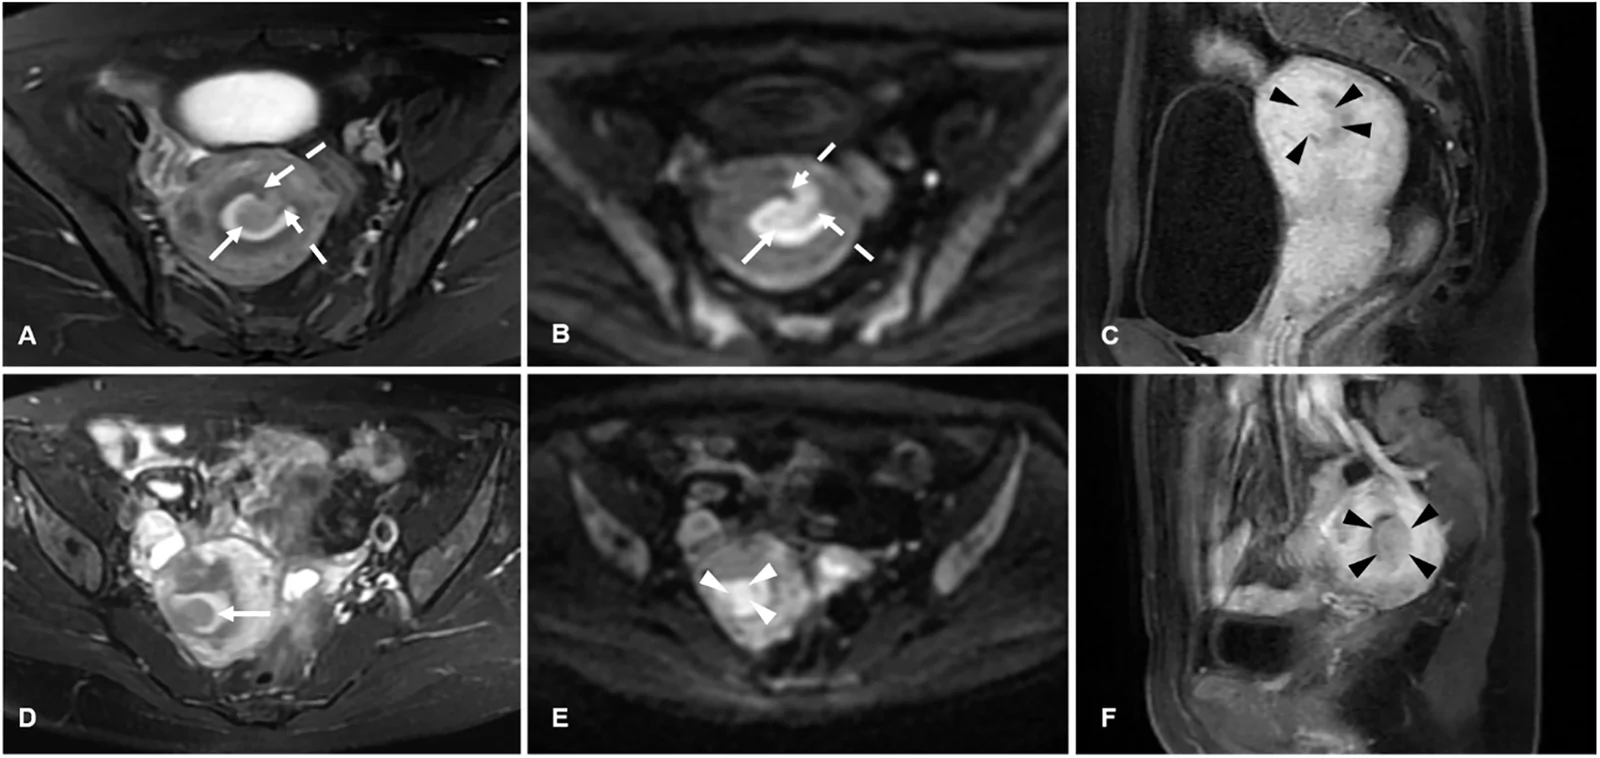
LG-ESS and pelvic magnetic resonance images from a 46-year-old (A–C) woman and a 54-year-old (D–F) woman. Axial T2W (A) and DW (B) images show a small nodular lesion protruding into the uterine cavity, appearing hyperintense on T2WI (relative to the uterine junctional zone) and hyperintense on DWI (arrows). Disruption of the adjacent junctional zone can be seen (dotted arrows). Sagittal T1-WI-enhanced scan (C) shows marked enhancement of the lesion (arrowheads). Axial T2W image (D) showing a T2WI mildly hyperintense nodule protruding into the uterine cavity (arrows), and axial DW image (E) showings lesion with a hyperintense signal (arrowheads) but with indistinct borders (consistent with the signal of the surrounding endometrium); a sagittal T1-WI-enhanced (F) image shows that the degree of lesion enhancement is slightly lower than that of the surrounding myometrium (arrowheads)
